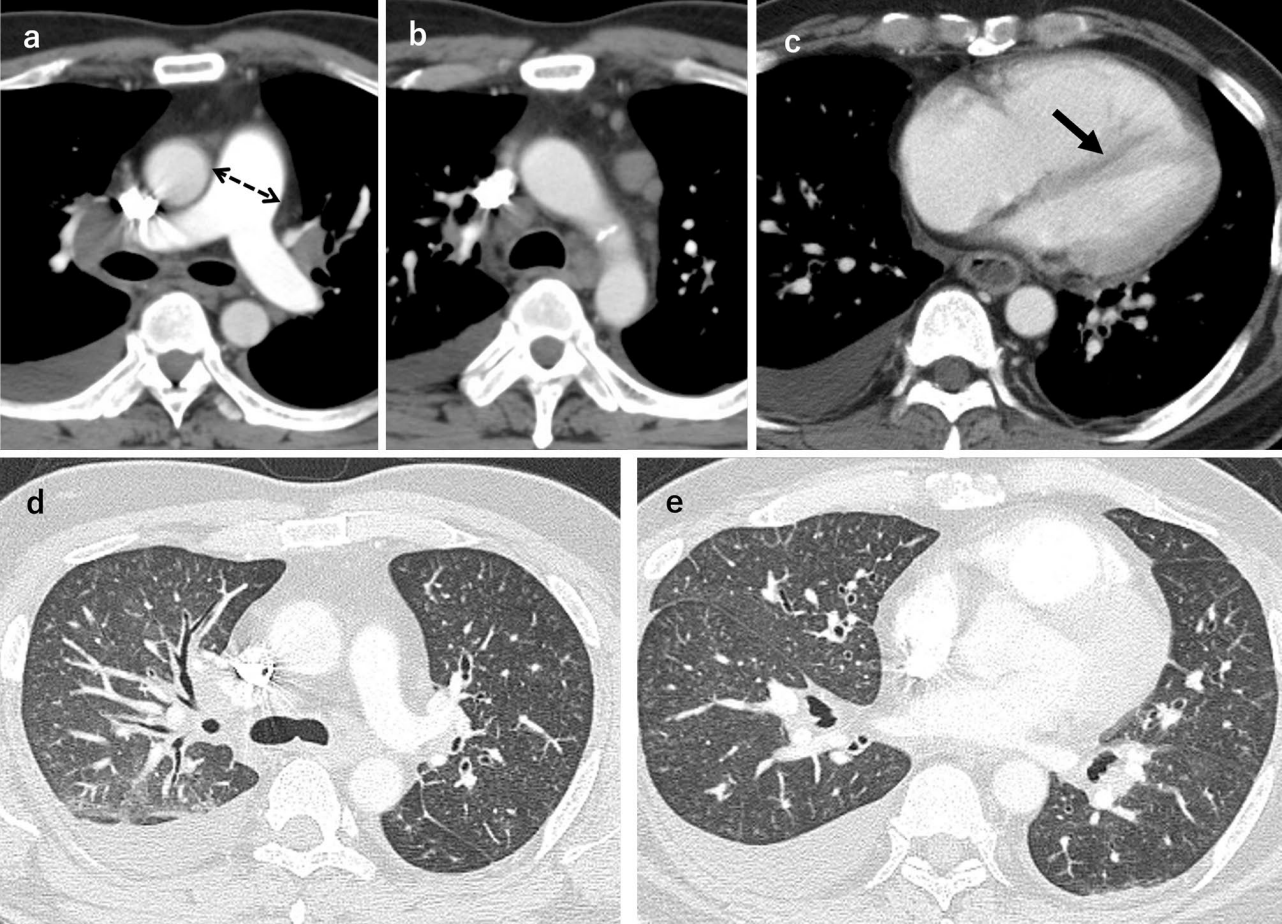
Pulmonary tumor thrombotic microangiopathy (PTTM). A man in his 40 s was diagnosed with PTTM secondary to a small gastric carcinoma at autopsy. Contrast-enhanced CT revealed pulmonary artery dilatation (a), mediastinal lymphadenopathy (b), right ventricular enlargement, leftward deviation of the interventricular septum and pleural effusion (c). The pulmonary findings included small centrilobular nodules, interlobular septal thickening, and thickening of the bronchovascular bundles (d–e)
